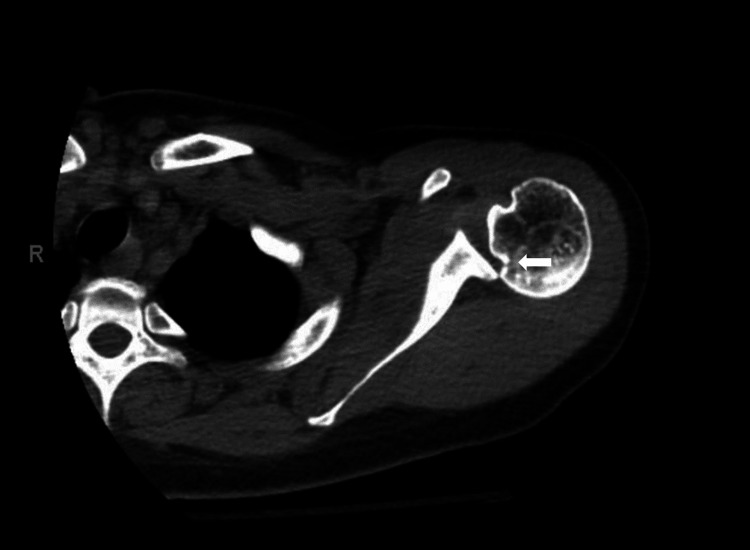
Computed tomography of the left shoulder demonstrating posterior shoulder dislocation and reverse Hill-Sachs deformity (white arrow)
